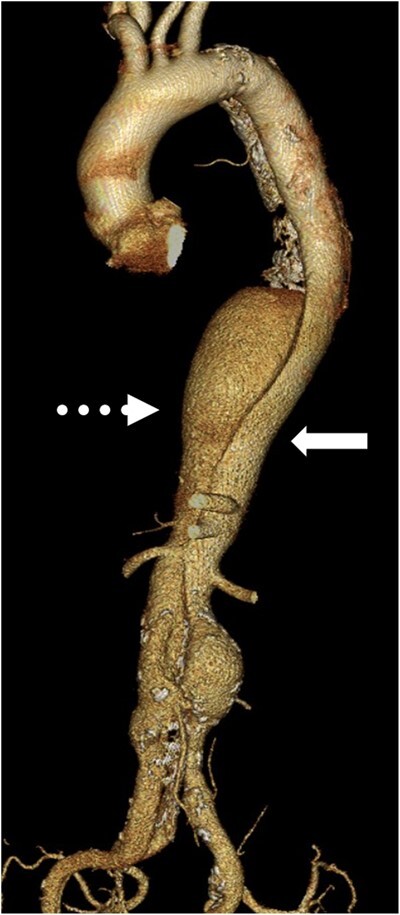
The preoperative computed tomography showing dissecting thoraco-abdominal aortic aneurysm with true lumen (solid arrow) and false lumen (dot arrow).
